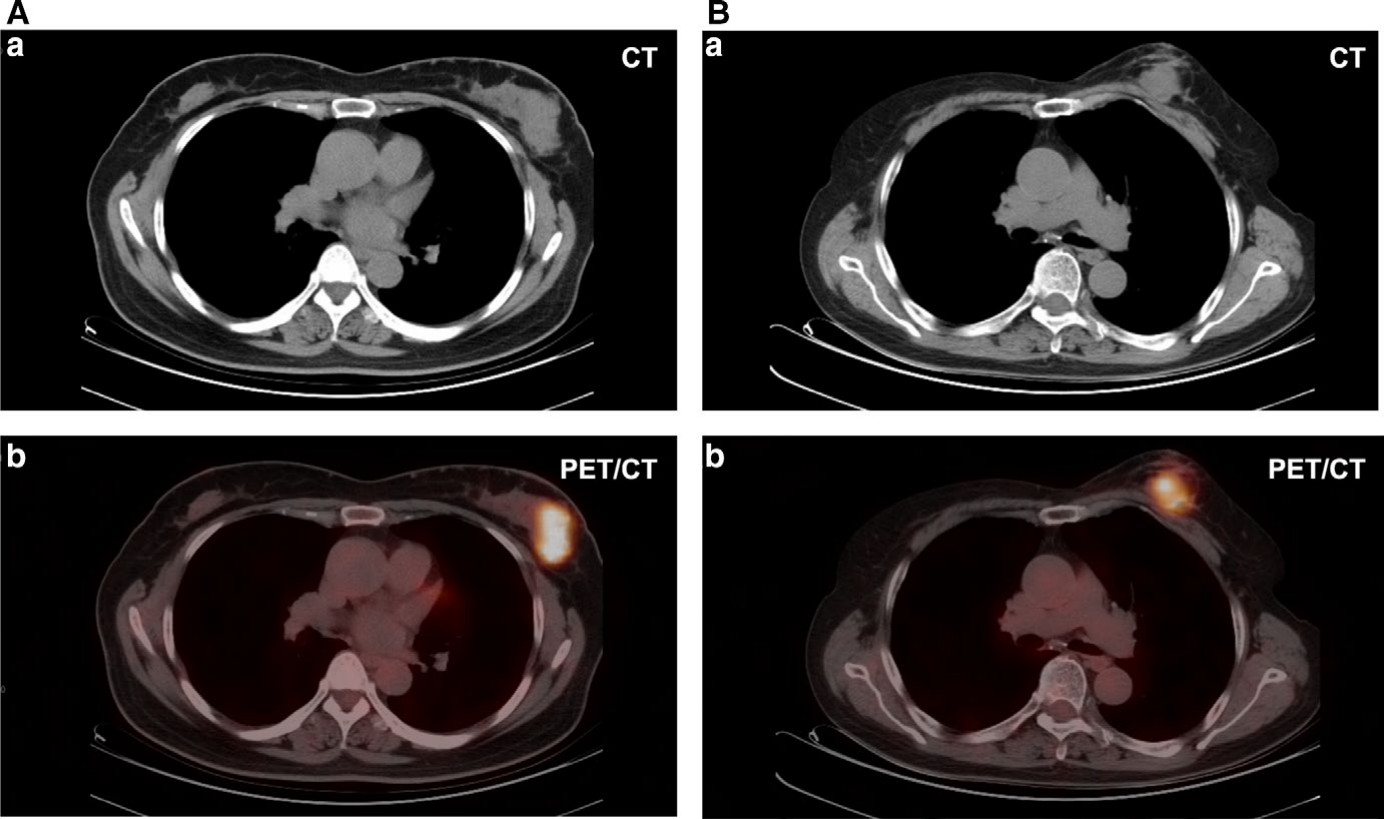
Two cases of CT and PET/CT images from patients with breast lymphoma (A) and breast carcinoma (B). CT, computed tomography; PET, positron emission tomography
